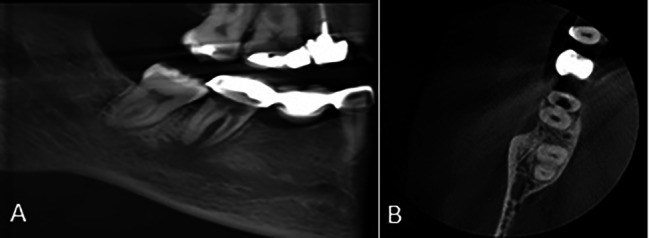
Mandibular second molar tooth with (A) type 2 morphology on the panoramic-like image and (B) double pattern on the axial CBCT section
